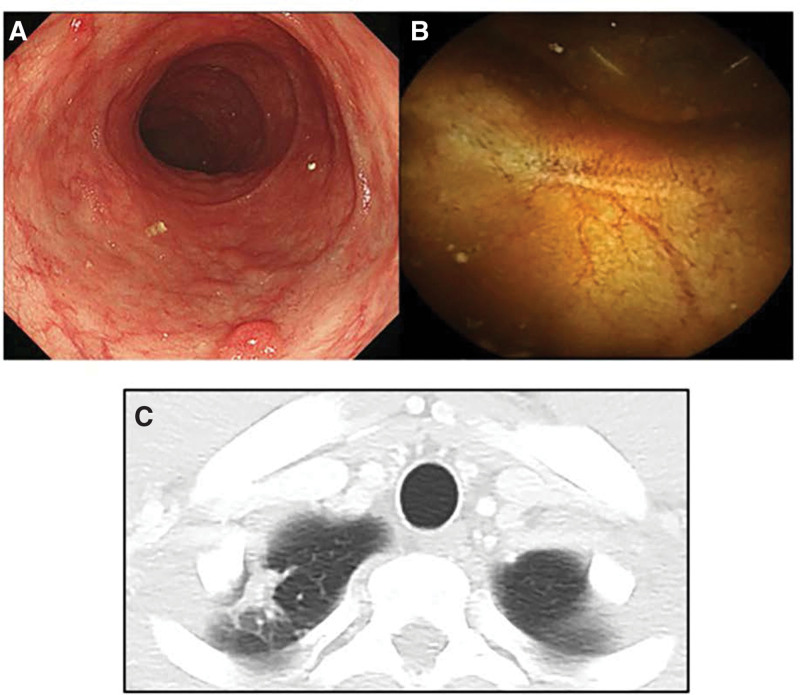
(A) Colonoscopy findings: mucosal healing in the terminal ileum 1 yr after VDZ administration. (B) Small bowel capsule endoscopic findings: mucosal healing in the whole small intestine 1 yr after VDZ administration. (C) Chest computed tomography findings: a shrunken nodular lesion 1 yr after VDZ administration. VDZ = vedolizumab.
